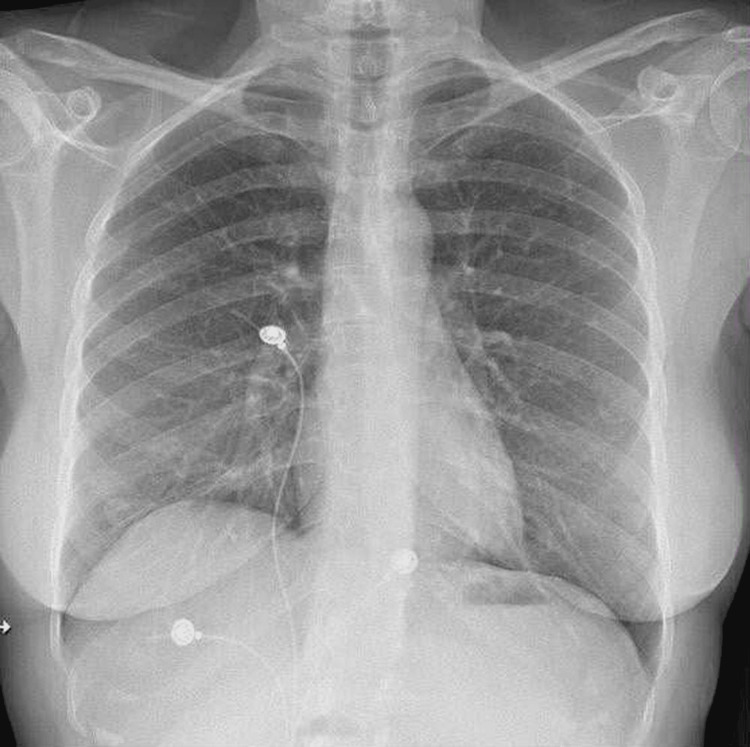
Chest X-ray showing no acute cardiopulmonary findings
